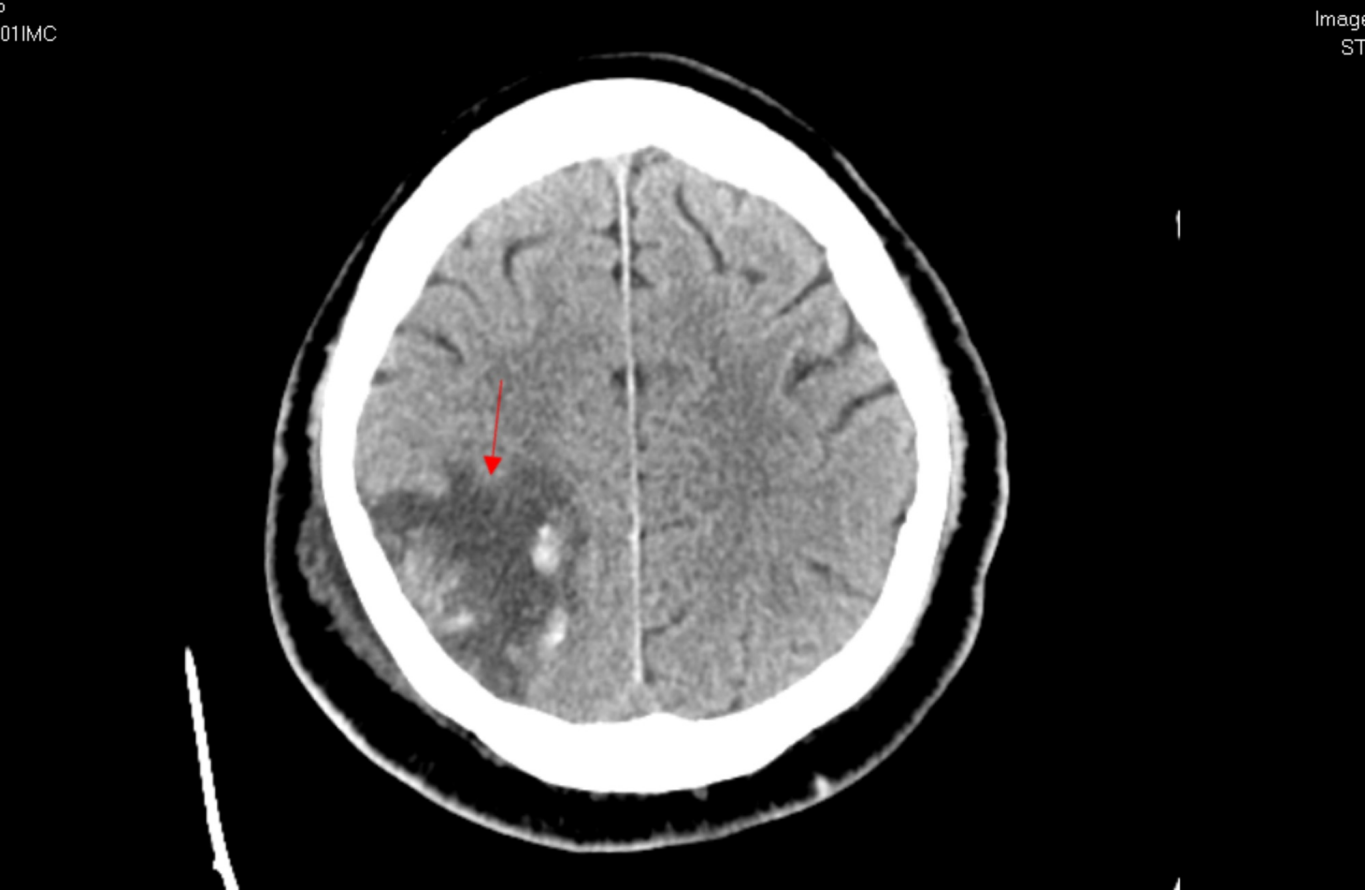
Case 2 head CT on April 22, 2020
